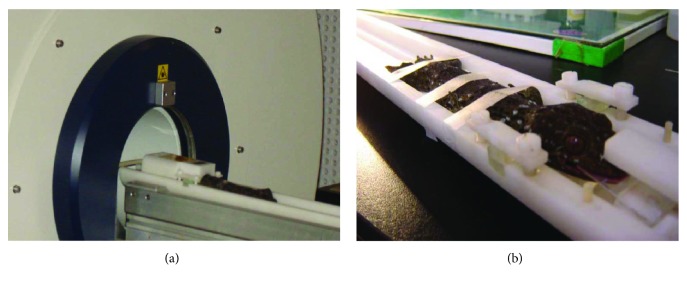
An anaesthetized gecko is placed into the MRI instrument, whose head and body are fixed to a custom-designed fixture (a). The close view of the fixed gecko in the fixture without cover (b).
